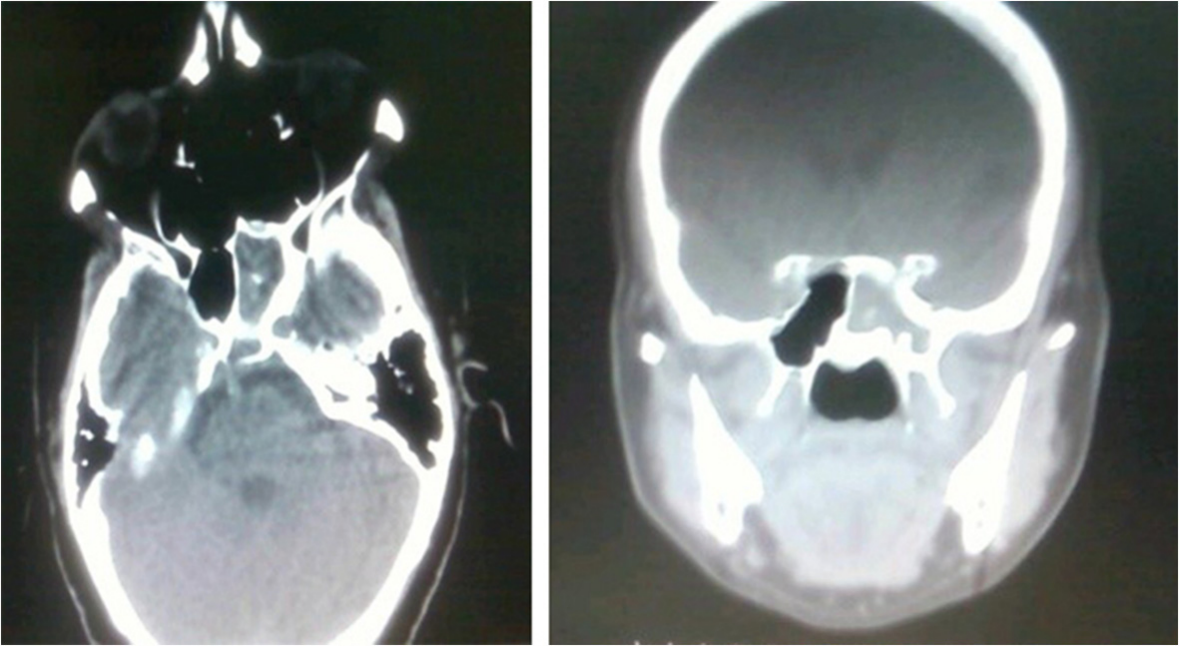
Patient’s CT scan of the paranasal sinuses. Opacification in the left sphenoid sinus with hypertrophic mucosa and secretions. Left: Axial view. Right: Coronal view.
